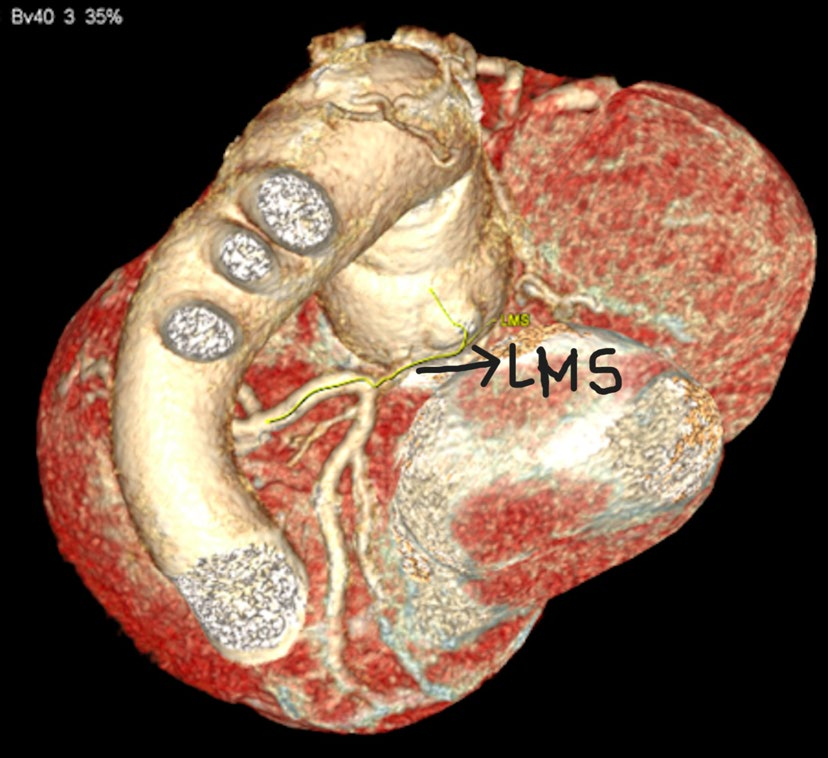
CT demonstrating the narrowing of the left main coronary artery
